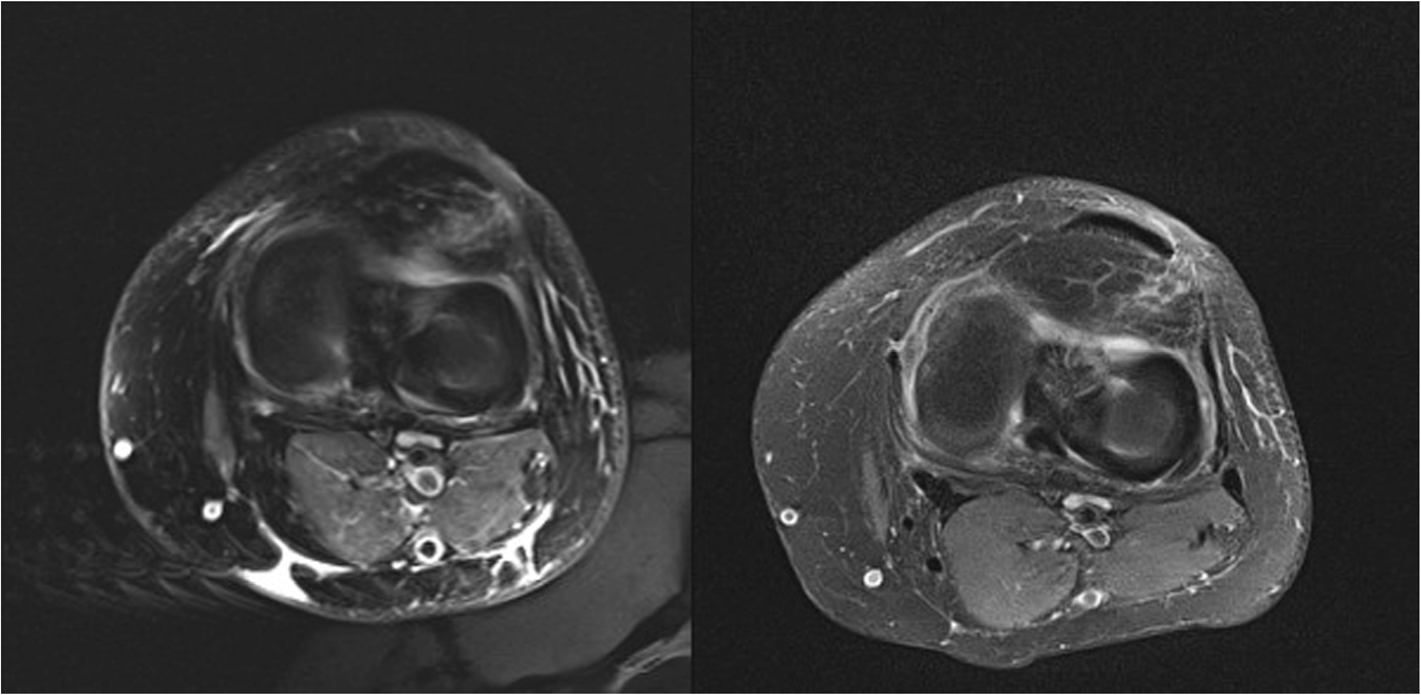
MRI after 30 (on the left) and 60 (on the right) days: the images show a reduction of imbibition and swelling of the periarticular tissues; besides the abscesses around the surgical site is not more detectable
